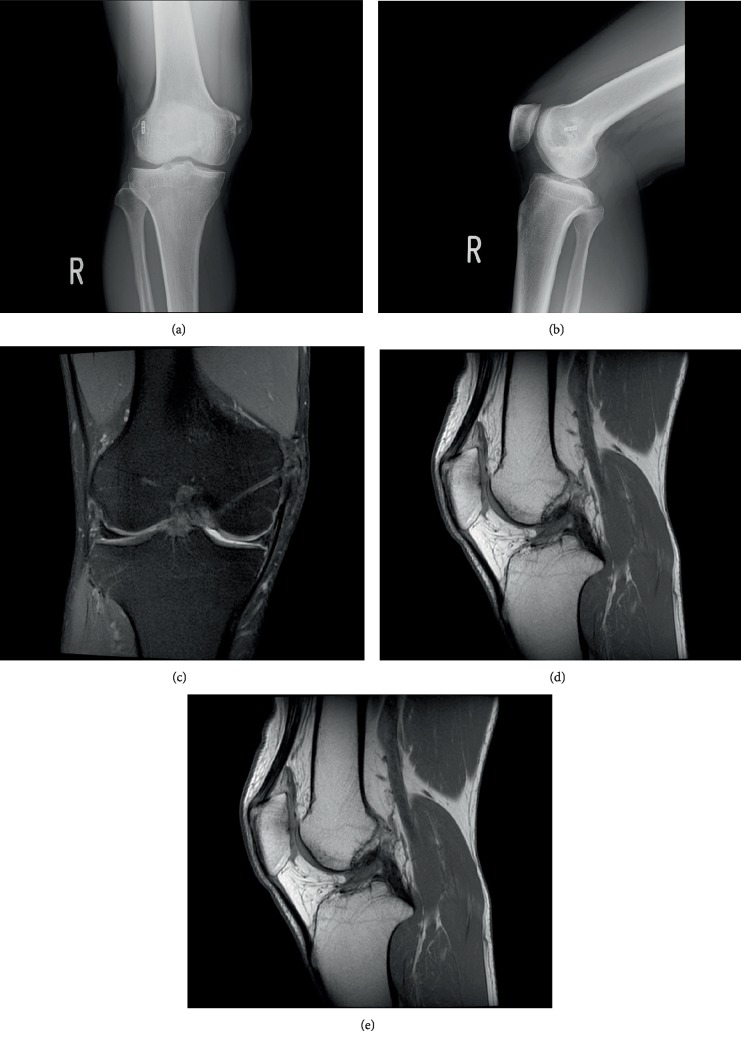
Images 2 years after surgery. (a, b) No progression of osteoarthritis seen on radiographs. (c–e) On MRI, the ACL, PCL, and MCL ligaments are in tension, and fiber continuity is maintained. ACL: anterior cruciate ligament; PCL: posterior cruciate ligament; MCL: medial collateral ligament; MRI: magnetic resonance imaging.
